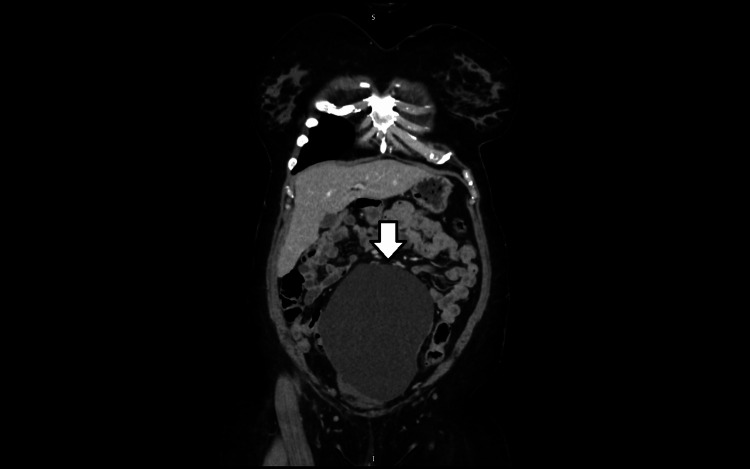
Coronal CT image showing a well-defined cystic lesion in the lower abdomen and pelvis. The lesion demonstrates homogeneous low attenuation without internal septations or solid components. Cross-sectional imaging demonstrated a large cystic lesion measuring approximately 19 cm in maximum dimension
